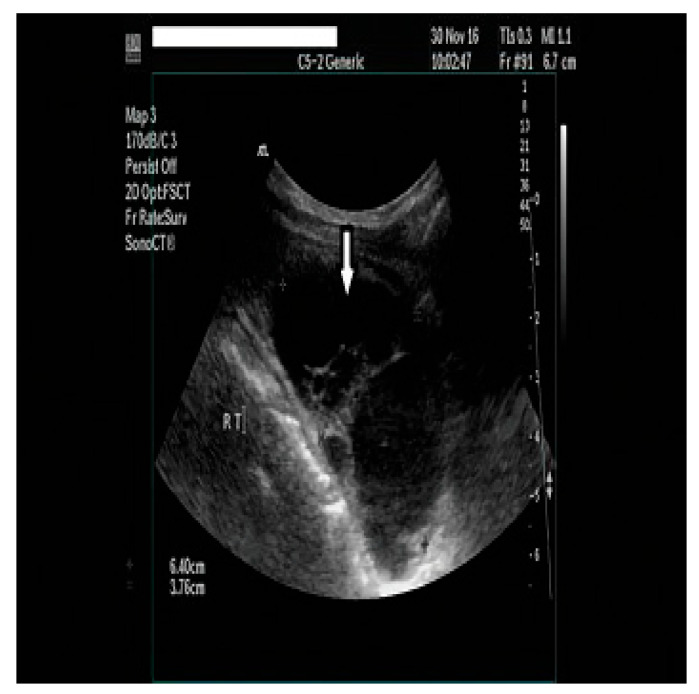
A neck ultrasonography (USG) showed a 6.90 × 3.05 × 2.60 cm multilobulated cyst in the lower part of the right thyroid lobe.
